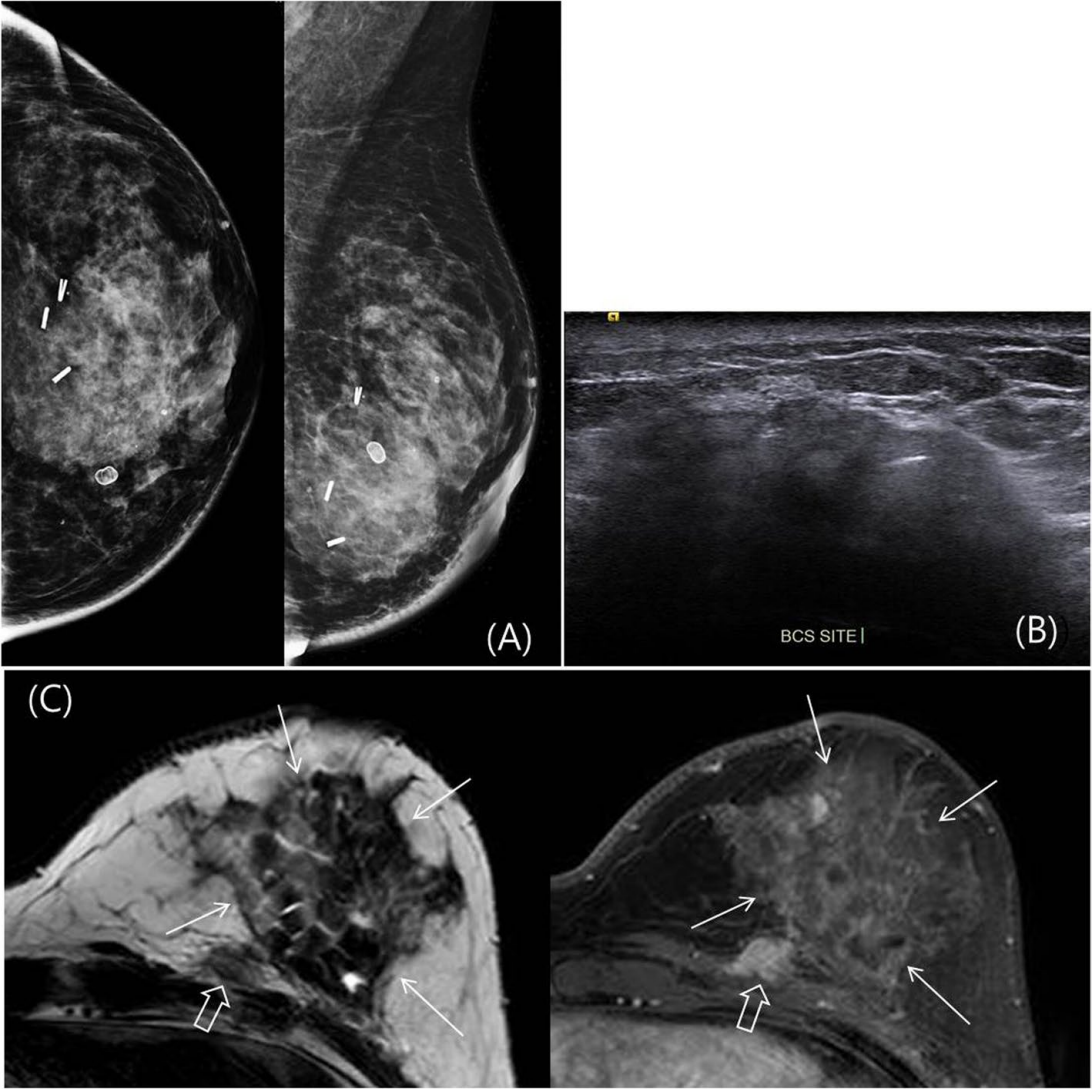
Follow-up imaging after 6 months of a 56-year-old woman who underwent oncoplastic BCS with ADM. A, B Post-surveillance mammogram and ultrasonogram were negative. C AB-MRI showed an irregular enhancing mass (block arrow) at the deep margin of the excision cavity filled with ADM (arrows). Second-look ultrasonography was performed, but the lesion at the deep portion was not identified due to shadowing. At surgery, a 0.7-cm-sized invasive cancer was confirmed
